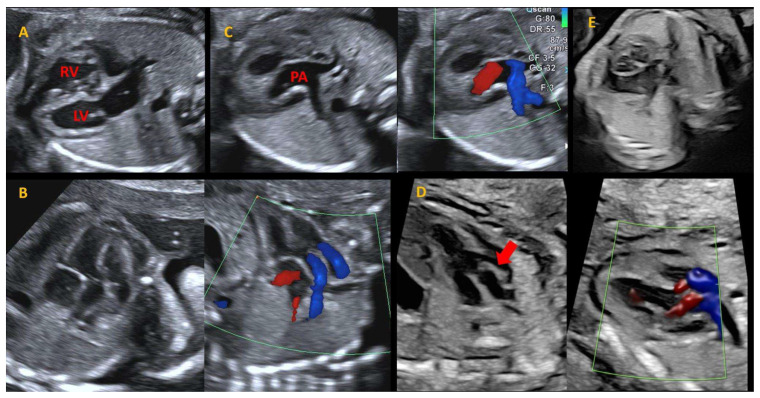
Fetal echocardiographic assessment in TGA through cranial sweep starting from 4CH going to the outflows. Panel (A): 4CH view, which appears normal in most fetuses with TGA; (B,C): outflow tracts’ assessment, showing parallel vessels arising from the 2 ventricles, with the posterior artery (PA), which bifurcates; (D): short-axis view, where both the semilunar valves appear in cross-sectional orientation; (E): the presence of a VSD should be investigated with 2D and color Doppler. In this panel, a mild posterior deviation of the conal septum is noted (red arrow).
